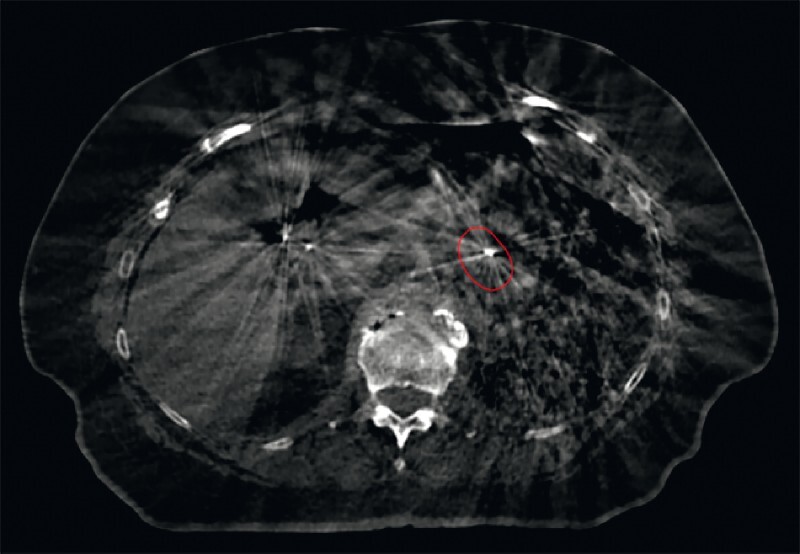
Cone-beam computed tomography (CBCT) image showing the fiducial marker implanted in the tumor of the pancreatic tail (encircled in red). The tumor itself is not visible, because of the low soft tissue contrast resolution of CBCT imaging; the radiopaque marker is essential for target volume localization. CBCT is performed before delivery of each radiation fraction to verify the target position.
